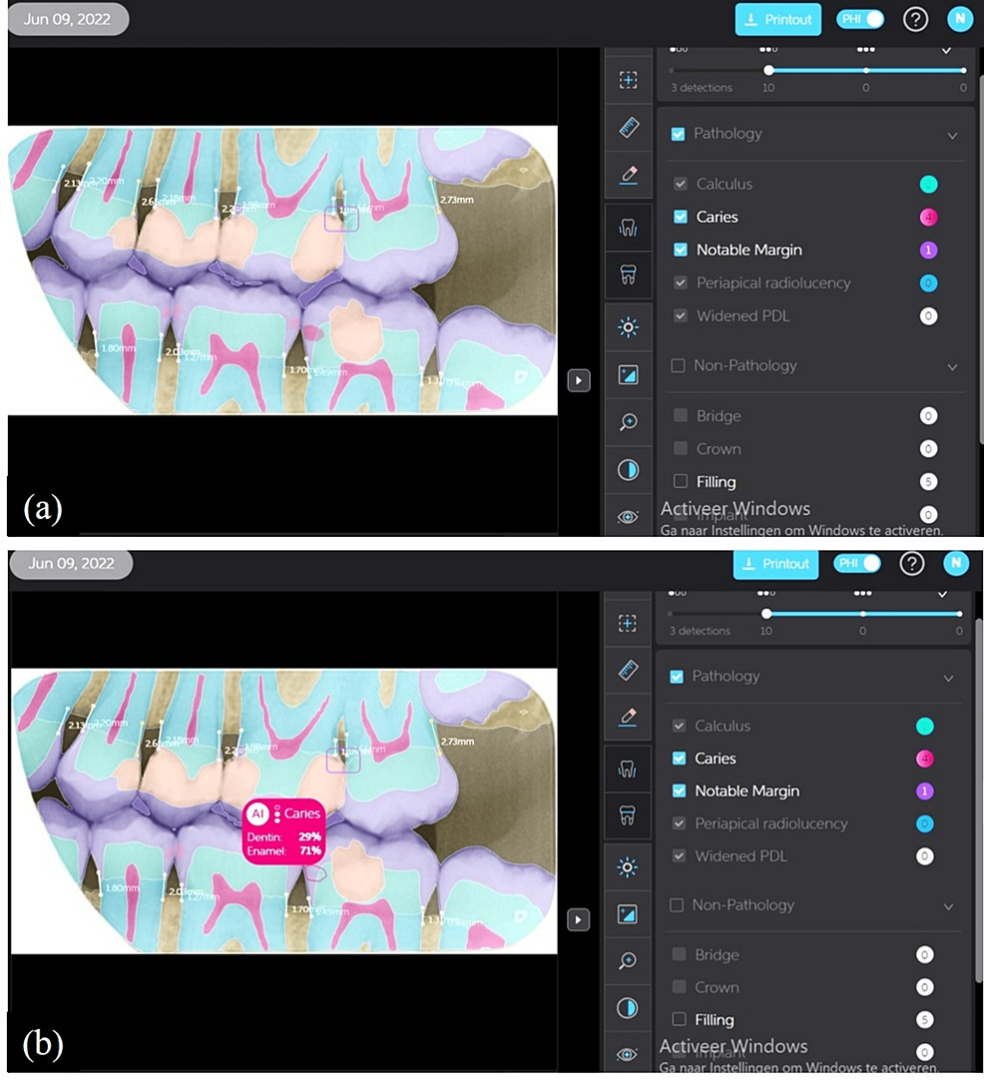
Role of artificial intelligence in the annotation of dental X-rays. (a) Segmentation of the different tooth structures and (b) details on the penetration level of the tooth decay. Image Credits: Dirk Neefs, DD-Care, Belgium
